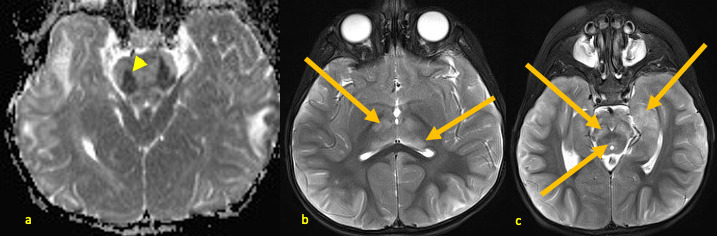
Now 30-month-old male with rAHNE. Brain MRI obtained during his second presentation for AHNE: axial ADC map (a) demonstrating low signal (arrowhead) in the pons; axial *T*
